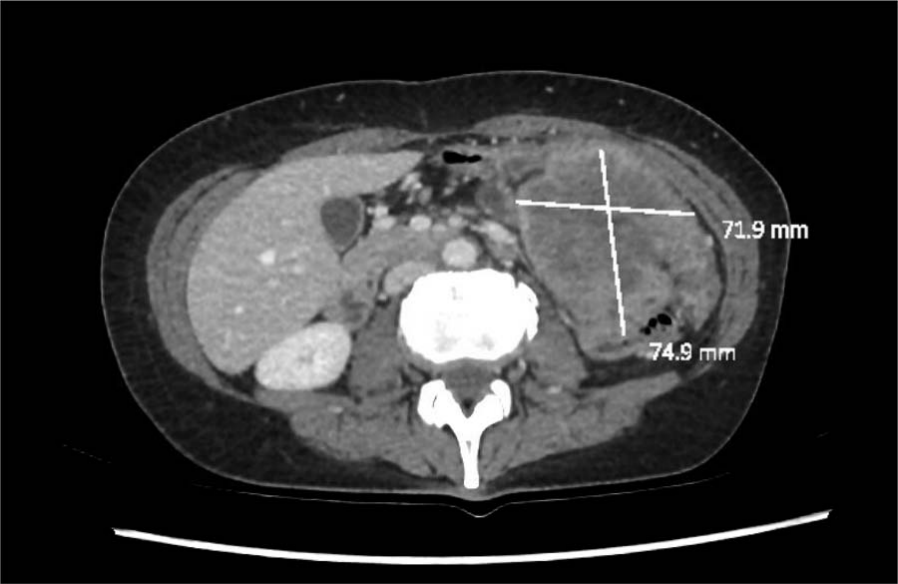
Axial slice of a CT demonstrating a large heterogenous mass in the left upper quadrant.
